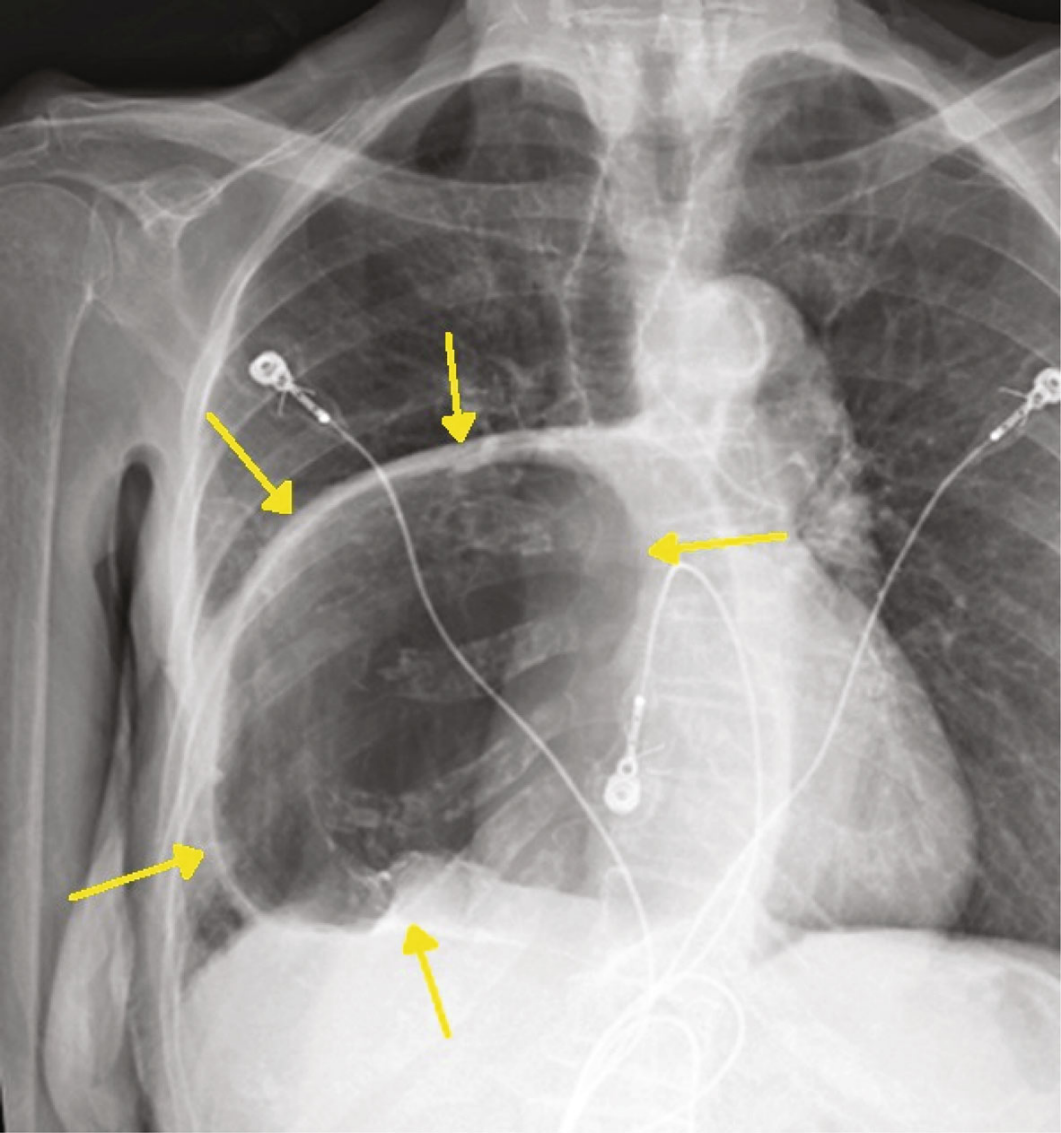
Upright chest X-ray showing large intrathoracic hiatal hernia between the yellow arrows.
